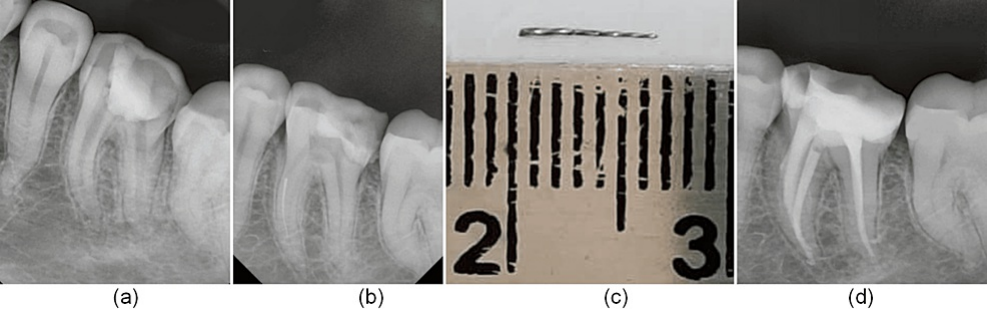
SI removal: (a) intraoral periapical showing deep restoration involving the pulp chamber. (b) Separated instrument in mesiobuccal canal of mandibular first molar. (c) Retrieved instrument fragment measured. (d) Intraoral periapical showing obturated mandibular molar.
